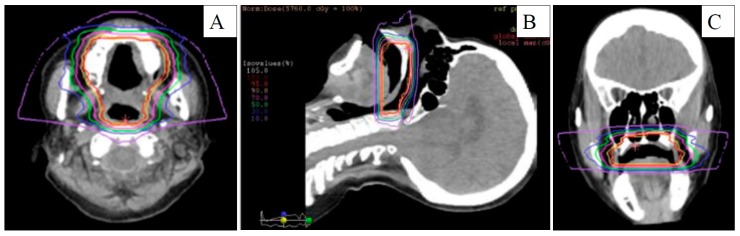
Computed tomography for carbon-ion dose distribution in initial mucosal melanoma. (A) Axial, (B) sagittal, and (C) coronal views. 70% dose: red-purple line, 50% dose: blue line, and purple line: 10%.
